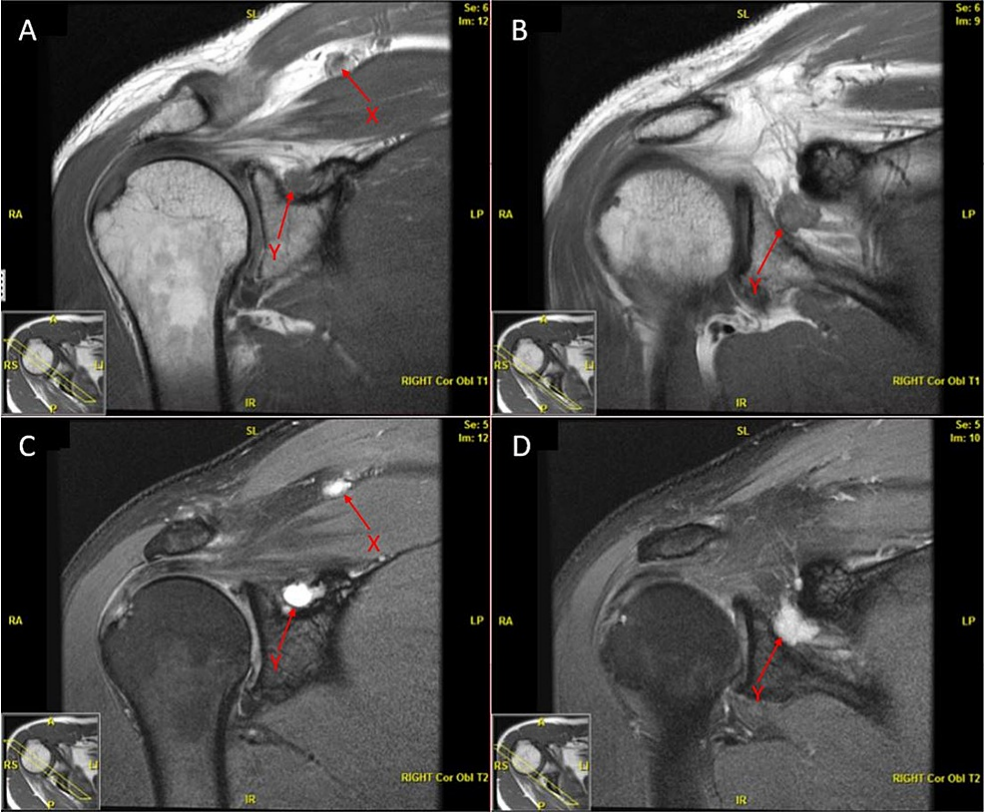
T1-weighted (A, B) and T2-weighted (C, D) MRI images of the right shoulder demonstrating mesenchymal tumors along the superior aspect of the supraspinatus muscle belly (arrow X) and within the suprascapular notch (arrow Y)
